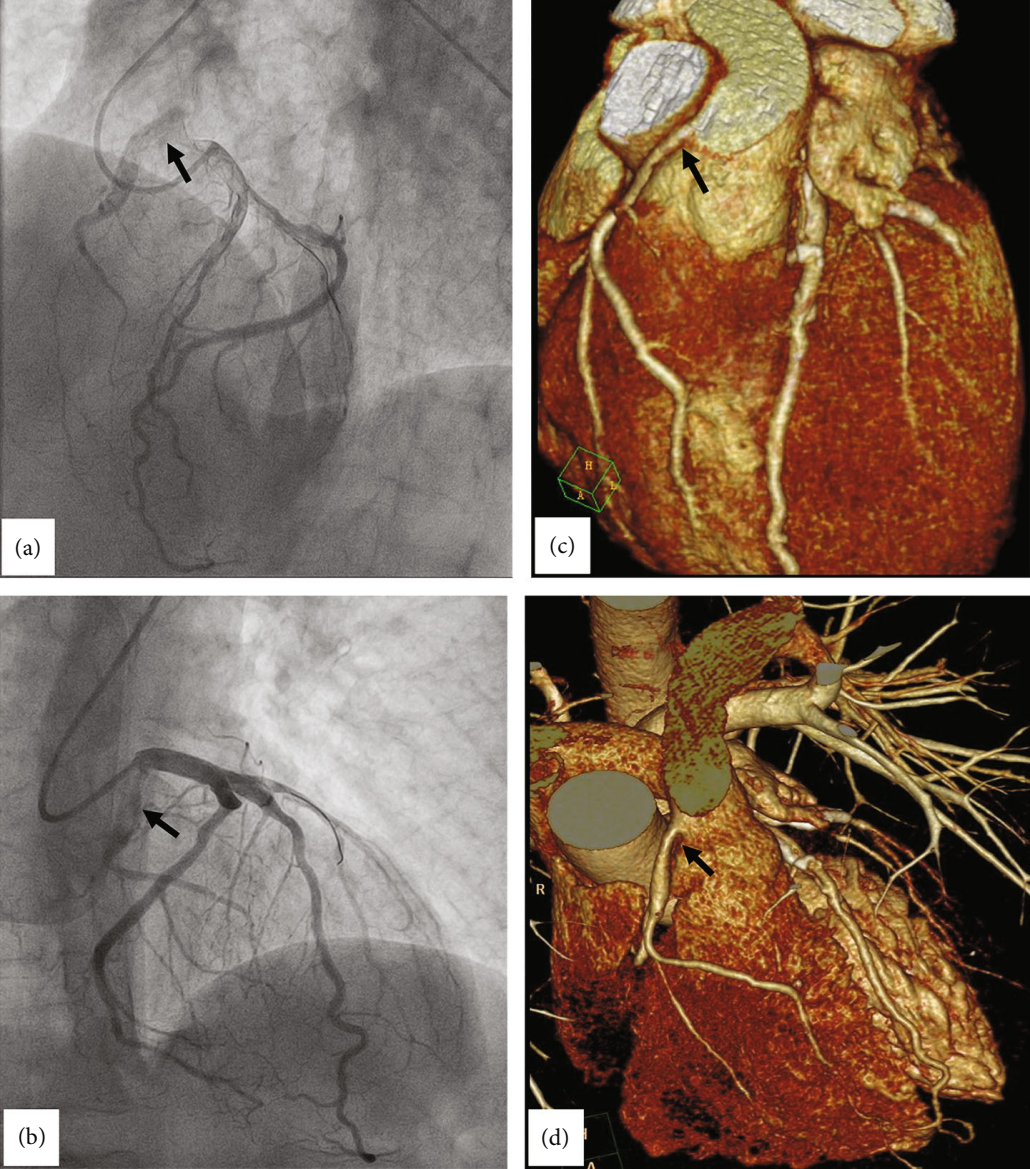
Coronary angiography, cranial views showing the retrograde filling of the right hypoplastic coronary artery toward the pulmonary artery (a, b). Corresponding projections from the computed tomography 3D reconstruction (the anomalous origin of the right coronary artery from the pulmonary artery is indicated by an arrow in c, d).
